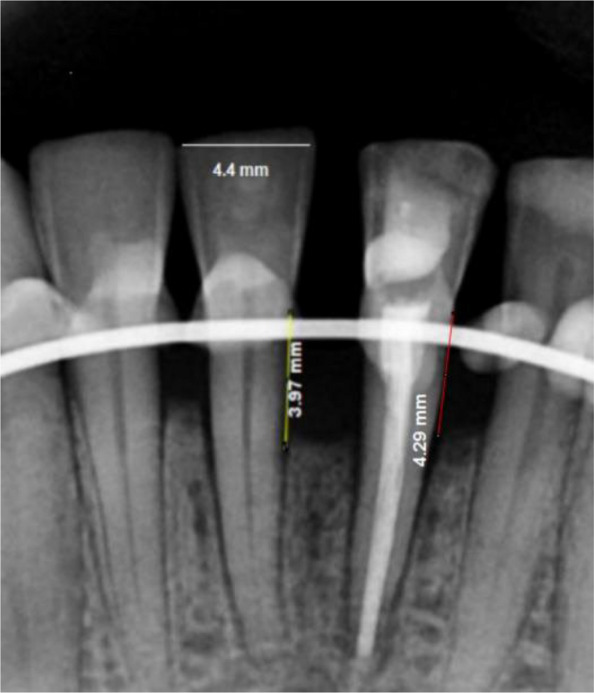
Post-operative radiographic measurements 6 months following therapy
